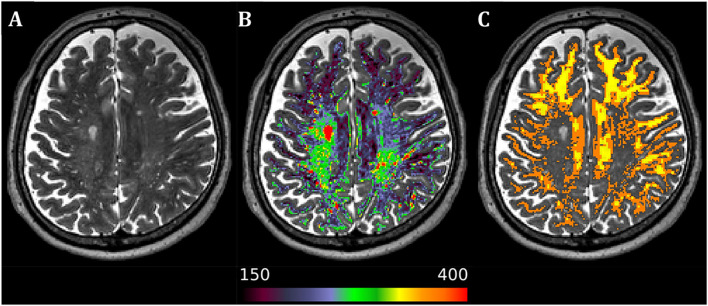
Axial image from a representative primary progressive multiple sclerosis subject. (A) Native T2-weighted image. (B) Superimposed white matter mask of the T2-weighted image with separate color palette (T2-weighted intensity range 150–400), allowing for easy detection of diffusely abnormal white matter and lesions. (C) Resulting normal-appearing white matter mask after manual visual validation and thresholding in 1-mm isotropic resolution of the original T2-weighted scan (orange) and 1.5-mm isotropic resolution of the diffusion-weighted image (yellow) after further inclusion probability thresholding at the level of 0.9 to avoid partial volume effects in lower resolution scans. Laterality conventions where the right side of the figure corresponds to the right side of the brain are used.
